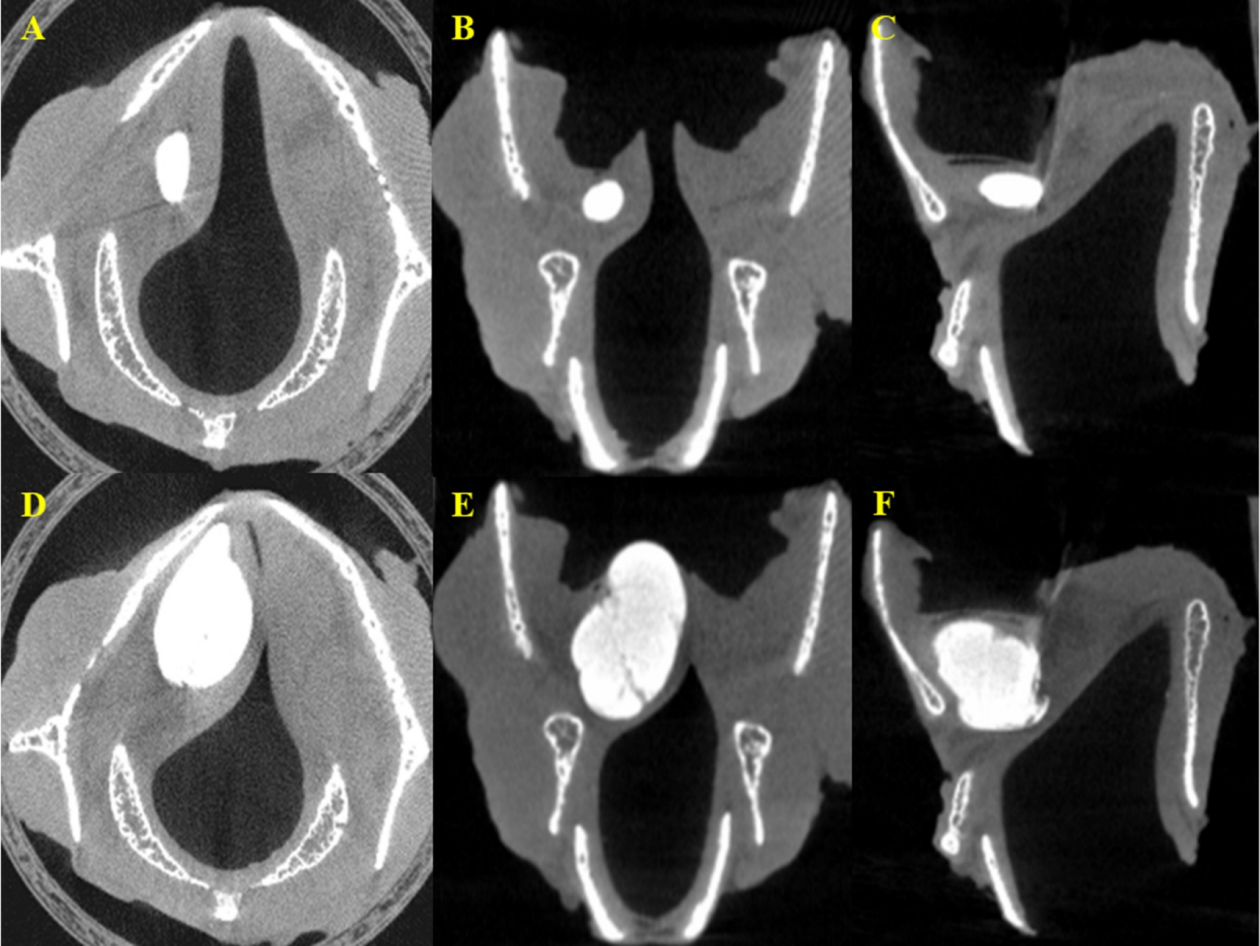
Serial view of micro–computed tomography. (A) Axial view at initial injection. (B) Coronal view at initial injection. (C) Sagittal view at initial injection. (D) Axial view before leakage. (E) Coronal view before leakage. (F) Sagittal view site before leakage.
